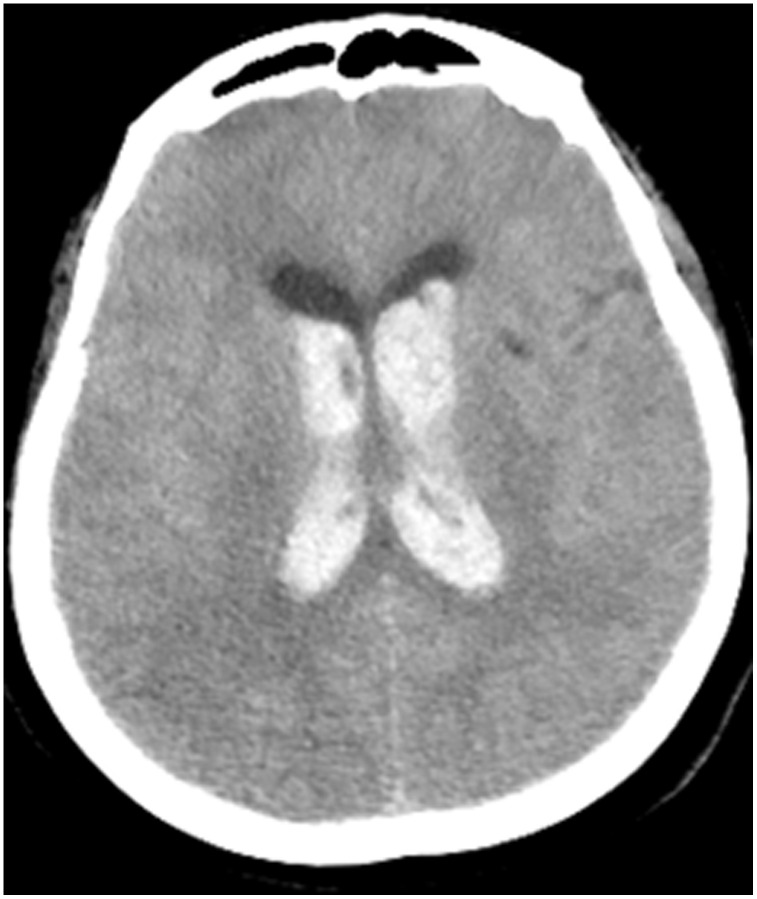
Case 3, head CT imaging: one patient had brainstem hemorrhage with ventricular cast and hydrocephalus.
